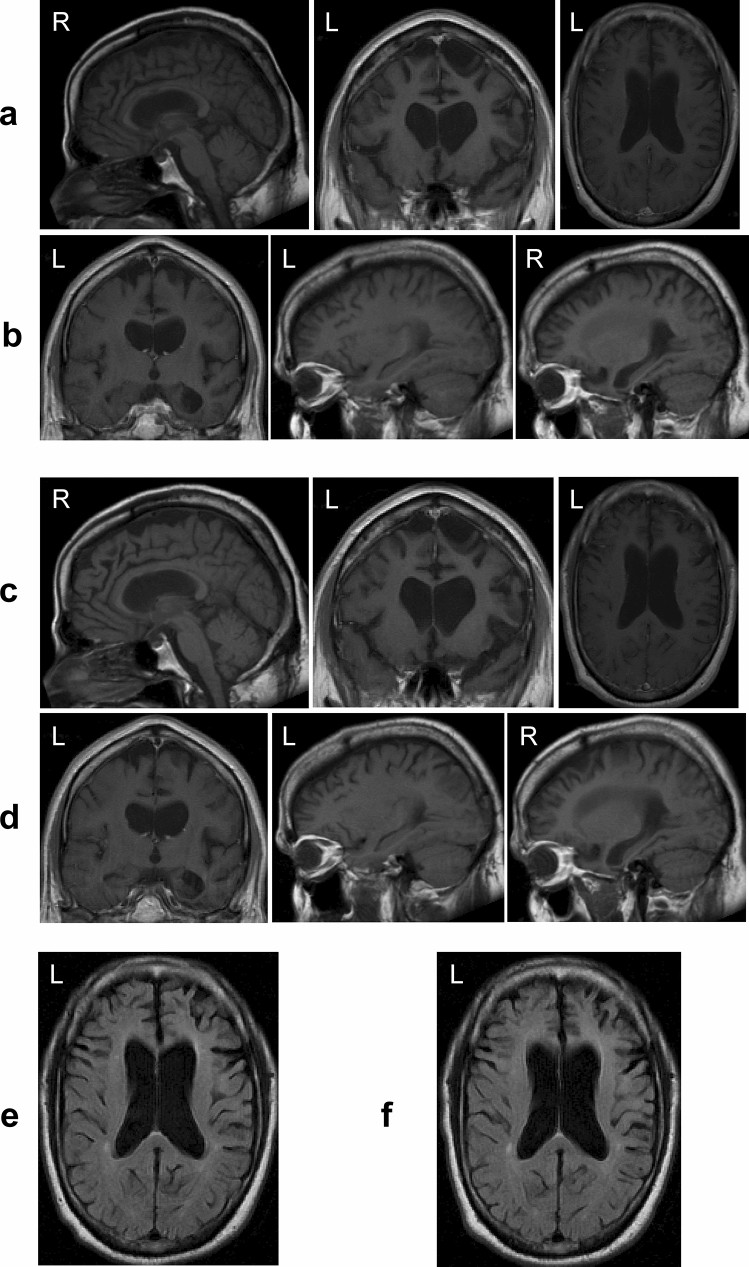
Comparison of two T1-weighted MRI scans of the brain of case 1 with *MAPT* mutation ∆K281. (a, b, e), Scan obtained during the first visit, when case 1 was 50 years old; (c, d, f), Scan obtained 5 months later. (a, c, e), Sagittal, coronal and axial MR images show atrophy of the frontal lobe with knife-blade atrophy of the gyri [middle panel in (a) and (c)], atrophy of the corpus callosum and severe enlargement of the lateral ventricles. (b, d, f), Coronal and parasagittal MR images show atrophy of the frontal, parietal and temporal lobes, as well as severe enlargement of the lateral ventricles. The MR images from the second visit reveal progression of the atrophy; differences in the dimensions of the lateral ventricles between first and second scans are particularly noticeable
